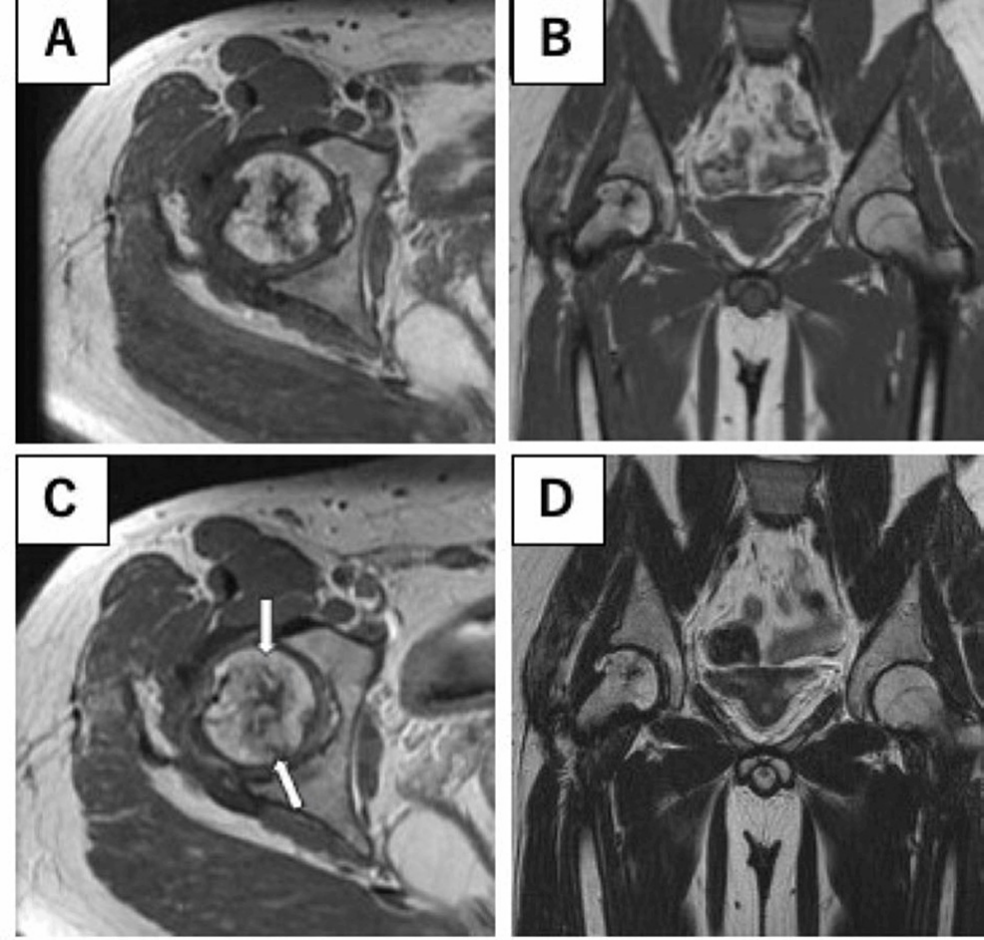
Magnetic resonance image taken 3.6 years postoperatively, revealing a greater contrast effect at the epiphysis (arrow). (A) Plain T1WI axial view; (B) plain T1WI coronal view; (C) gadolinium-enhanced T1WI axial view; (D) plain T2WI coronal view.
